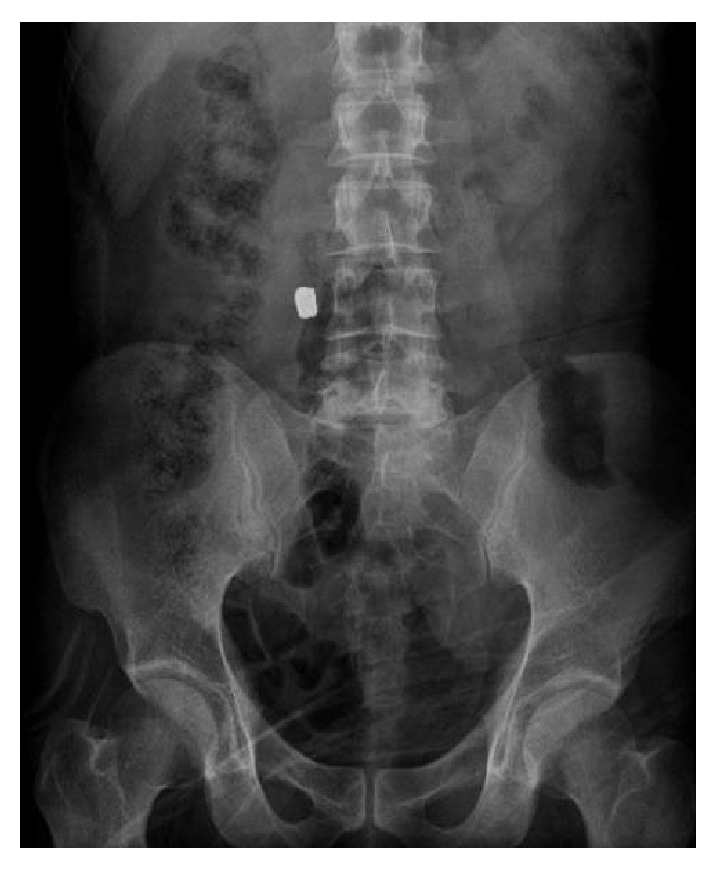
Bullet seen on the anterior-posterior radiograph.
